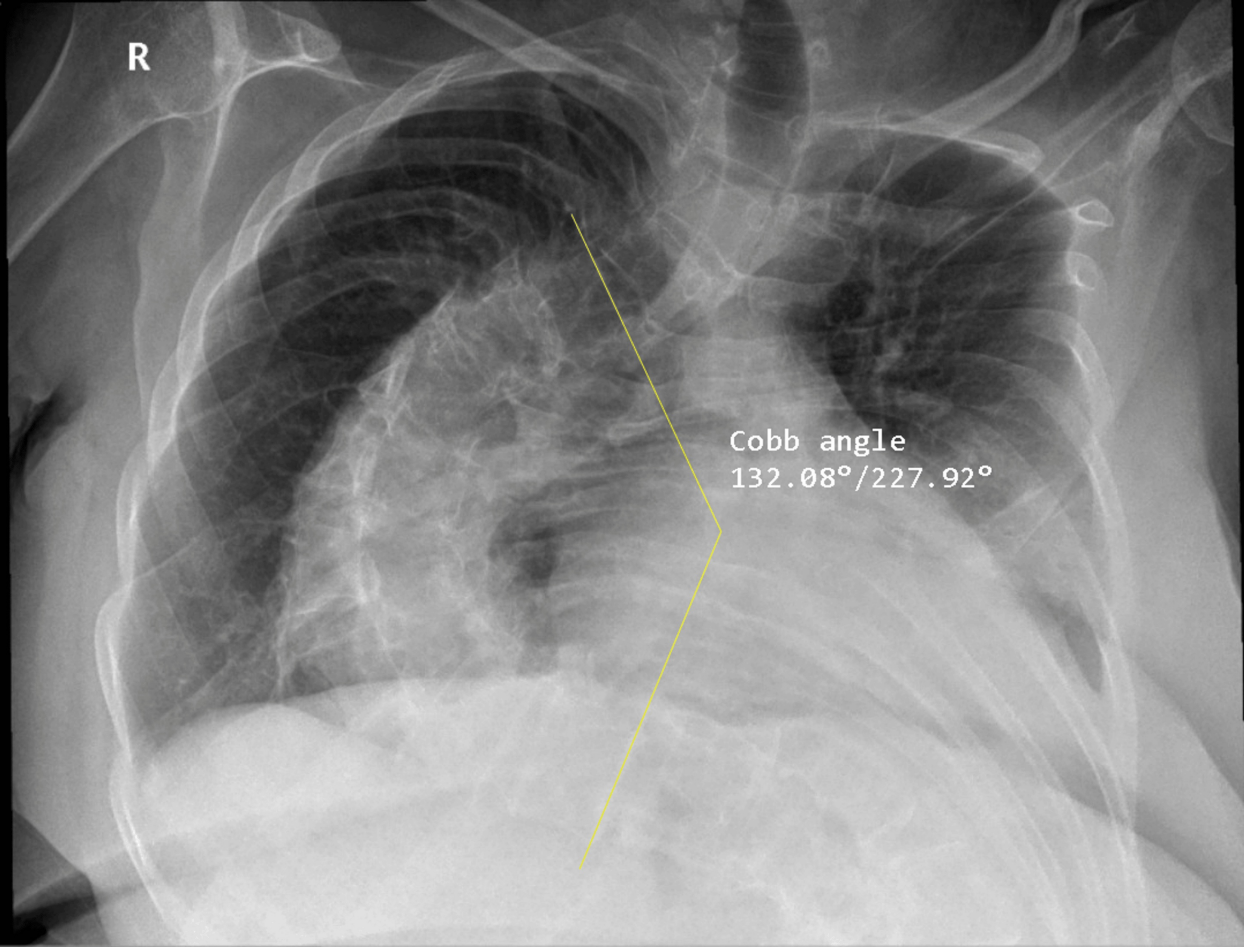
Chest radiograph. Anteroposterior view demonstrating a marked S‑shaped thoracolumbar scoliosis with right‑sided thoracic convexity. The Cobb angle measures 130°, consistent with very severe scoliosis.
